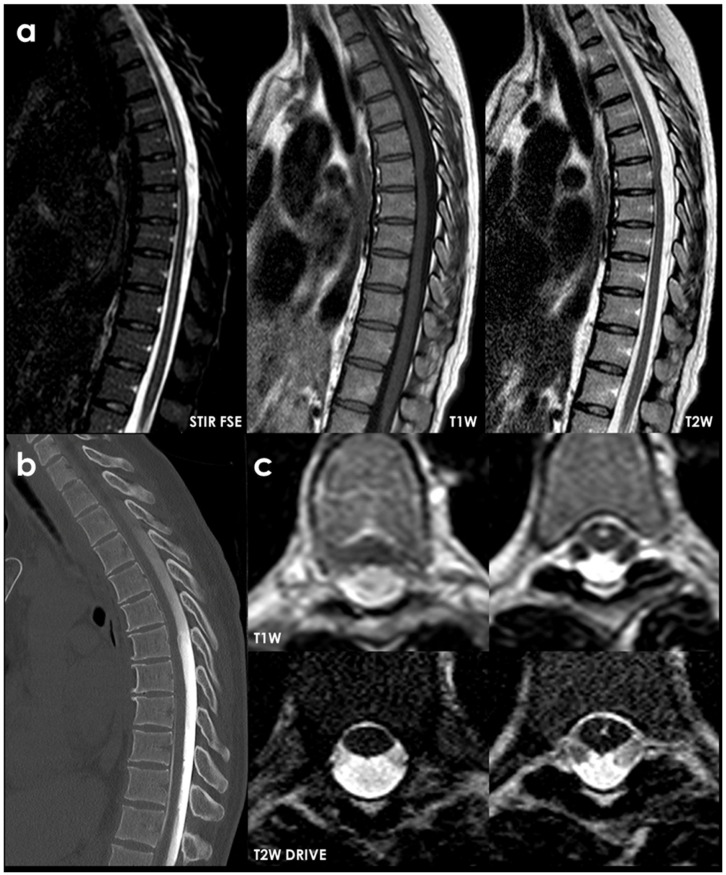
Spinal cord herniation in sagittal dorsal MRI (a) with the focal ventral attraction of the spinal cord at a vertebral body level, in the corresponding sagittal CT myelography (b) confirming the lack of contrast at the ventral side of the spinal cord at the level of attraction, and, finally, axial MRI (c) at the attraction level (left images) and under just this level (right images).
